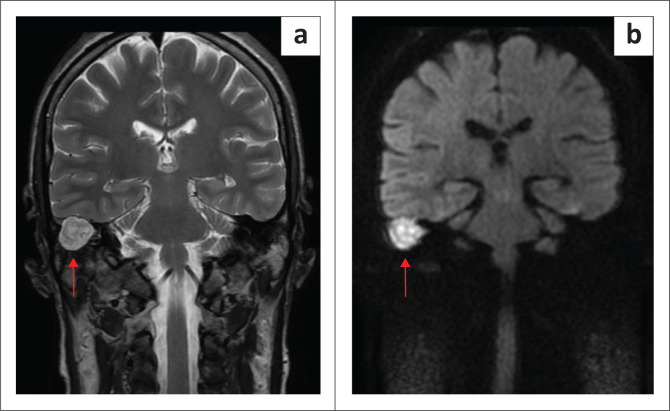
Right ear cholesteatoma in a 26-year-old female patient. (a) Coronal T2-weighted MRI and (b) coronal non-echo planar diffusion-weighted MRI images demonstrate the lesion, which shows increased T2 signal intensity and corresponding restricted diffusion consistent with cholesteatoma (red arrows).
